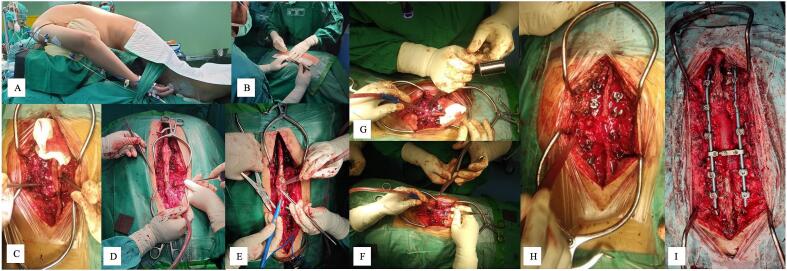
The intraoperative picture of surgical technique: (A) radiographic plain x-ray of the pelvis; (B) posterior approach; (C, D) pedicle screw insertion; (E, F) laminectomy decompression; (G) osteotomies procedure; (H) performing reduction with IOM; (I) posterior fixation.
